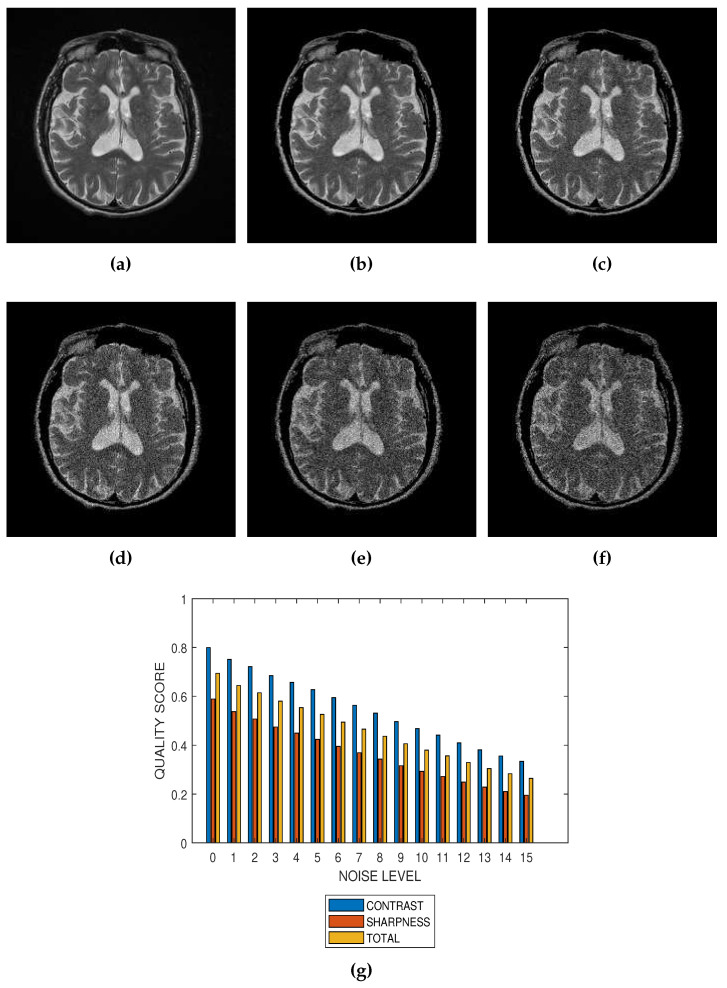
(a) A T2 weighted MRI slice from ADNI and its degraded versions at Rician noise levels (b) 3 percent, (c) 6 percent, (d) 9 percent, (e) 12 percent and (f) 15 percent. (g) Variation of contrast, sharpness and total quality scores for Rician noise levels that vary from 0 percent to 15 percent.
